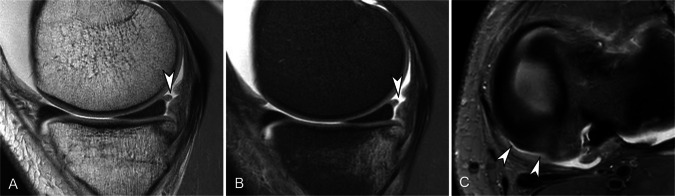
Meniscocapsular junction injury in a 25-year-old man who sustained ACL rupture while playing basketball. Sagittal proton-density-weighted MRI (A), sagittal T2-weighted fat-suppressed MRI (B), and axial proton-density-weighted fat-suppressed MRI (C) demonstrate full thickness longitudinal-vertical tear at the meniscocapsular junction of the posterior horn of the medial meniscus (arrowheads)
